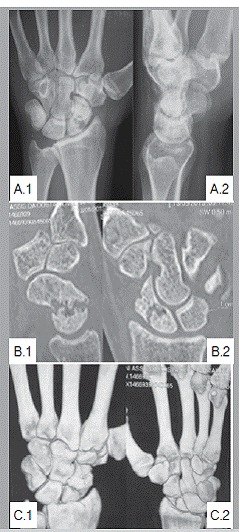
(A1 and A2) Preoperative anteroposterior and profile x-ray of the wrist (patient A) showing scaphoid nonunion, with no signs of arthrosis or Lichtmann grade 1 carpal instability; (B1 and B2) Sagittal and coronal sections Computed Tomography with good delimitation of bone resorption of nonunion focus without major angular change. (C1 and C2) 3D Reconstruction images of the wrist.
